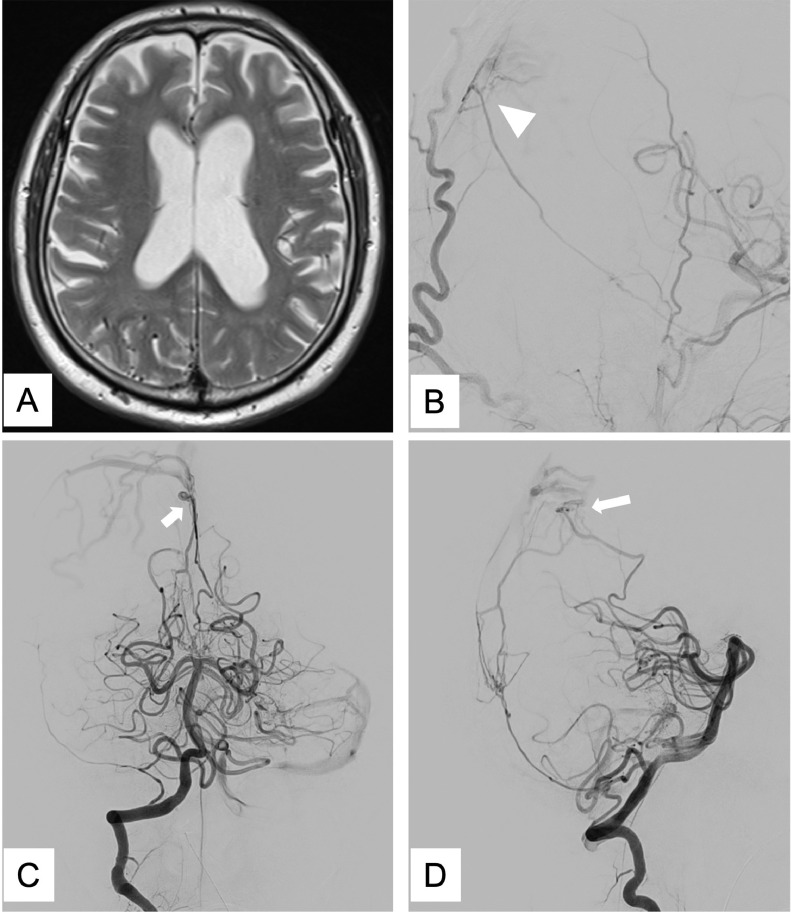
T2-weighted imaging showing the dilatation of cortical veins at the right occipital lobe (A). The right external carotid angiography image of the lateral view showing the arteriovenous fistula on the falx (arrowhead) (B). Right vertebral angiography showing a similar fistula (arrow) (C, D). C: anteroposterior view, D: lateral view
